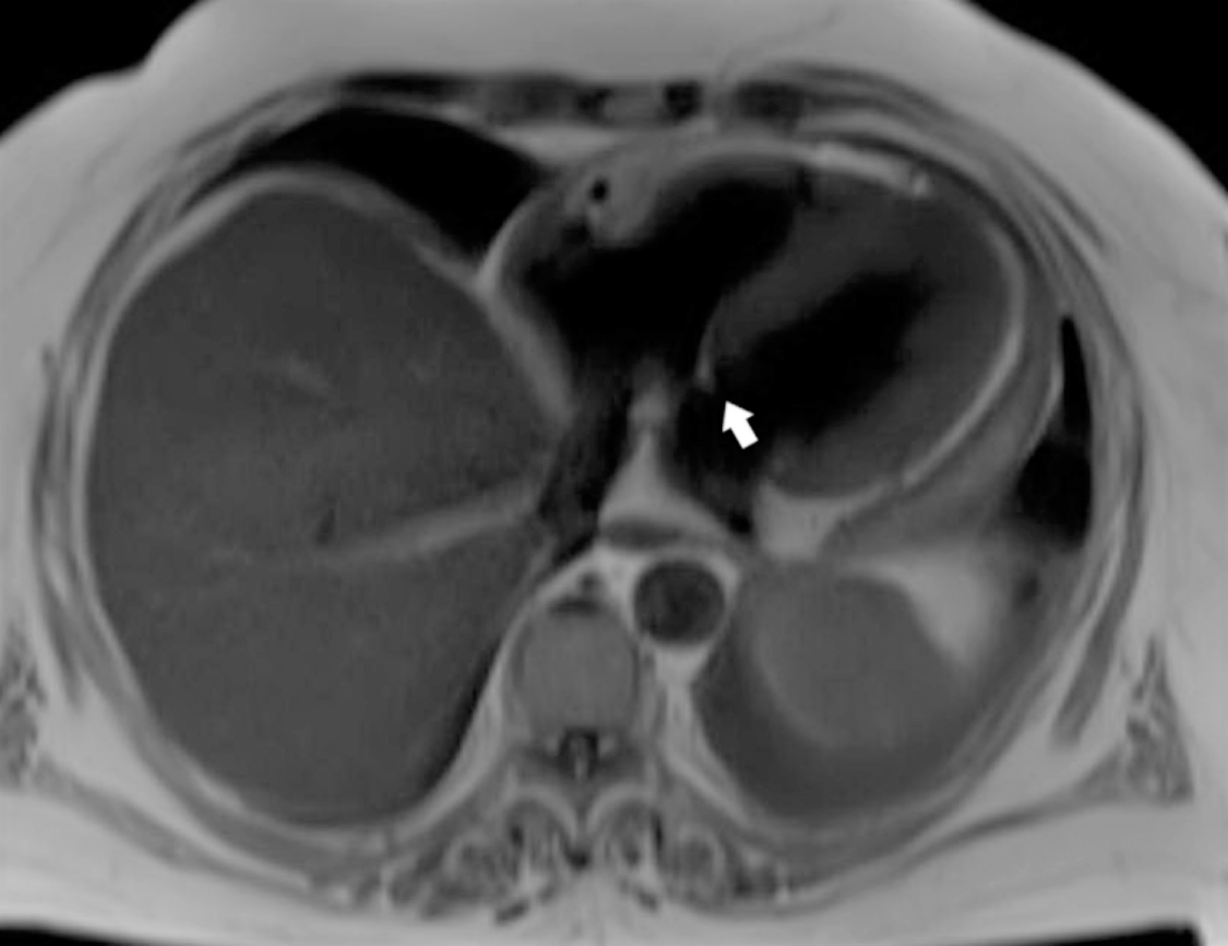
Cardiac magnetic resonance imaging (MRI) showing basal septal mid-wall enhancement of non-ischemic type consistent with myocarditis or cardiomyopathy
